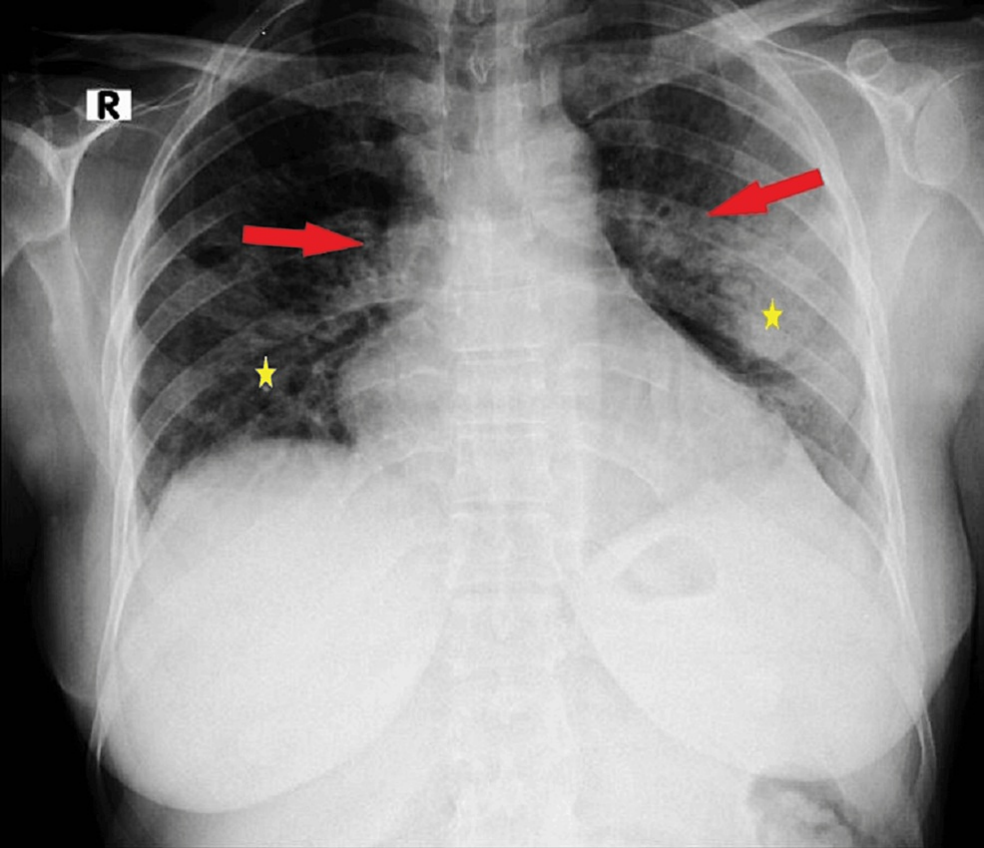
Chest X-ray X-ray demonstrates bilateral perihilar infiltration (stars) and a distinctive "flask-shaped" cardiac silhouette (arrows).
